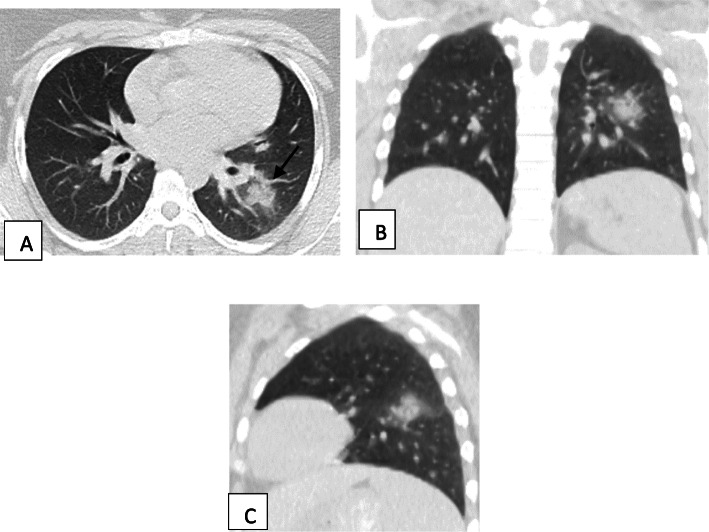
Unenhanced chest CT of a 29-year-old female patient with COVID-19. a An axial lung window image shows left lower lobe central broncho-vascular patch of consolidation [arrow]. b Same finding on the coronal and c sagittal reformatted images. CT score is 2
